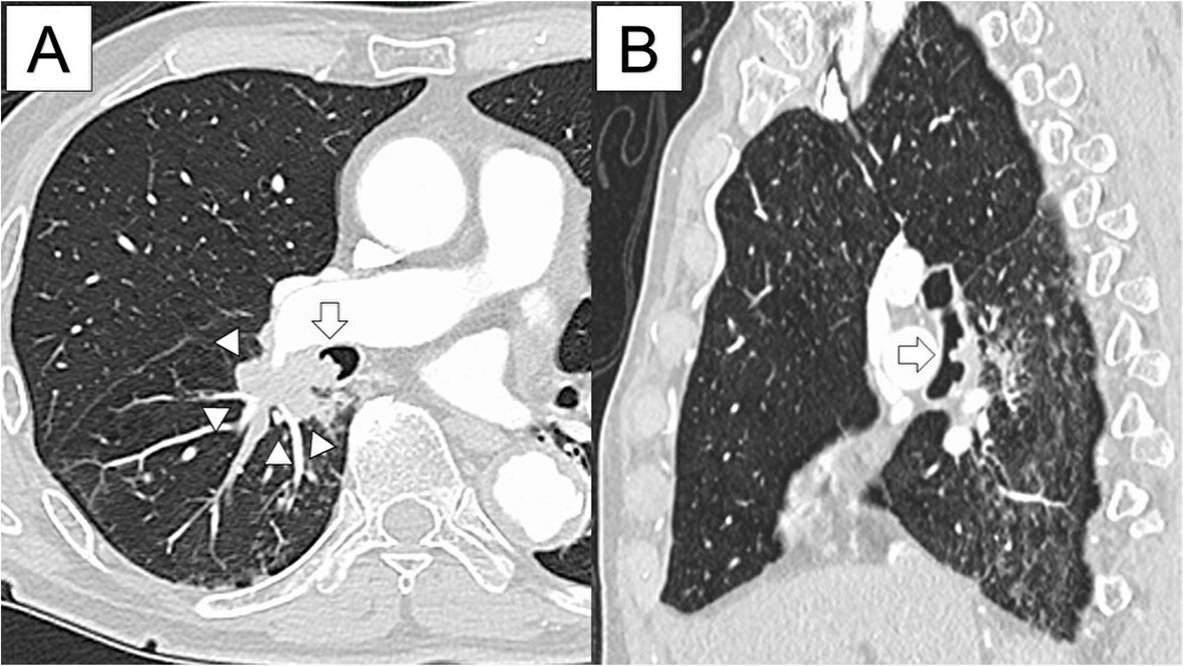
Computed tomography (A transverse view, B sagittal view) showing a tumor in the right lung S6 (arrowhead), extending continuously from B6 to bronchus intermedius (arrow). The peripheral lung at S6 shows nodular opacities due to obstructive pneumonia
